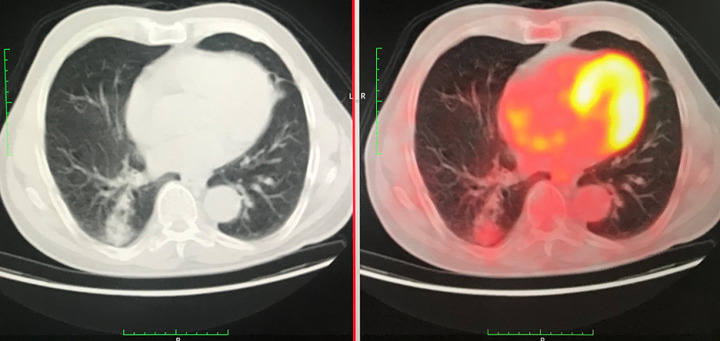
comparison of images taken by PET-CT and CT
